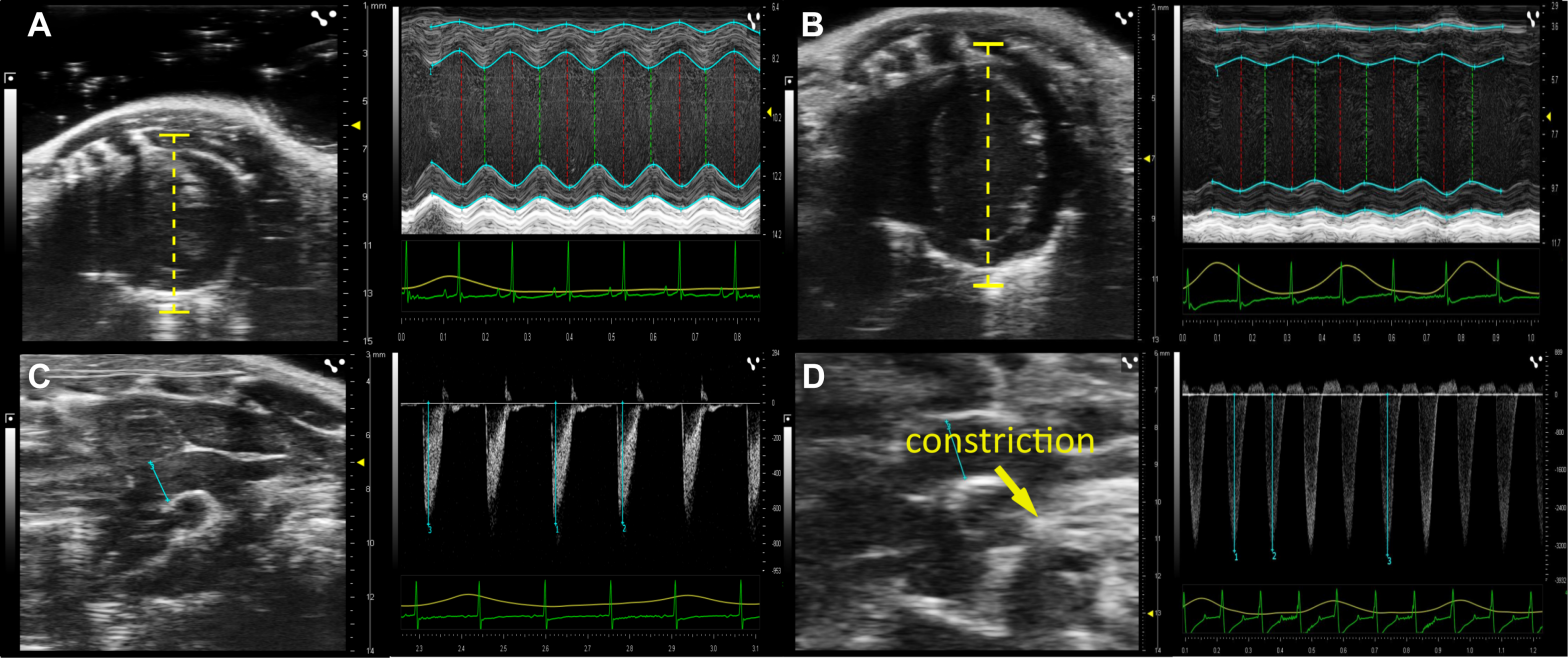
Development of heart failure after TAC operation. Original echocardiographic images before and 12 weeks after TAC. (A) Short Axis View (SAX) of the left ventricle (left image) and line scan (right image) measuring wall thickness and heart volume during systole and diastole before TAC surgery (time point 0) and (B) 12 weeks post TAC surgery. (C) Aortic Arch View (AA; left image) and pulsewave doppler velocity measurement of the transverse aortic arch (in mm/s) before TAC surgery and (D) 12 weeks post TAC surgery.
